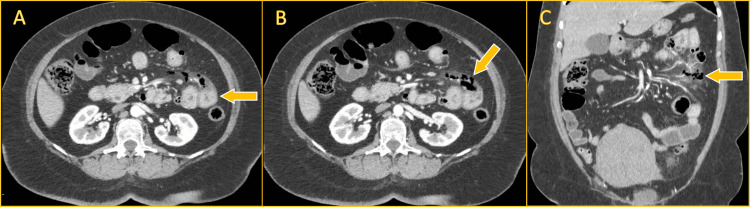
Contrast-enhanced abdominal CT demonstrating findings consistent with complicated jejunal diverticulitis (A) Axial view showing segmental wall thickening of a jejunal loop with multiple diverticula (arrow), indicative of an inflamed segment. (B) Axial view demonstrating adjacent extraluminal gas (arrow) and mesenteric fat stranding, consistent with localized perforation. (C) Coronal view confirming the presence of extraluminal air (arrow) adjacent to the affected jejunal segment, along with surrounding inflammatory changes. An incidental large uterine myoma is also visible in the pelvis.
